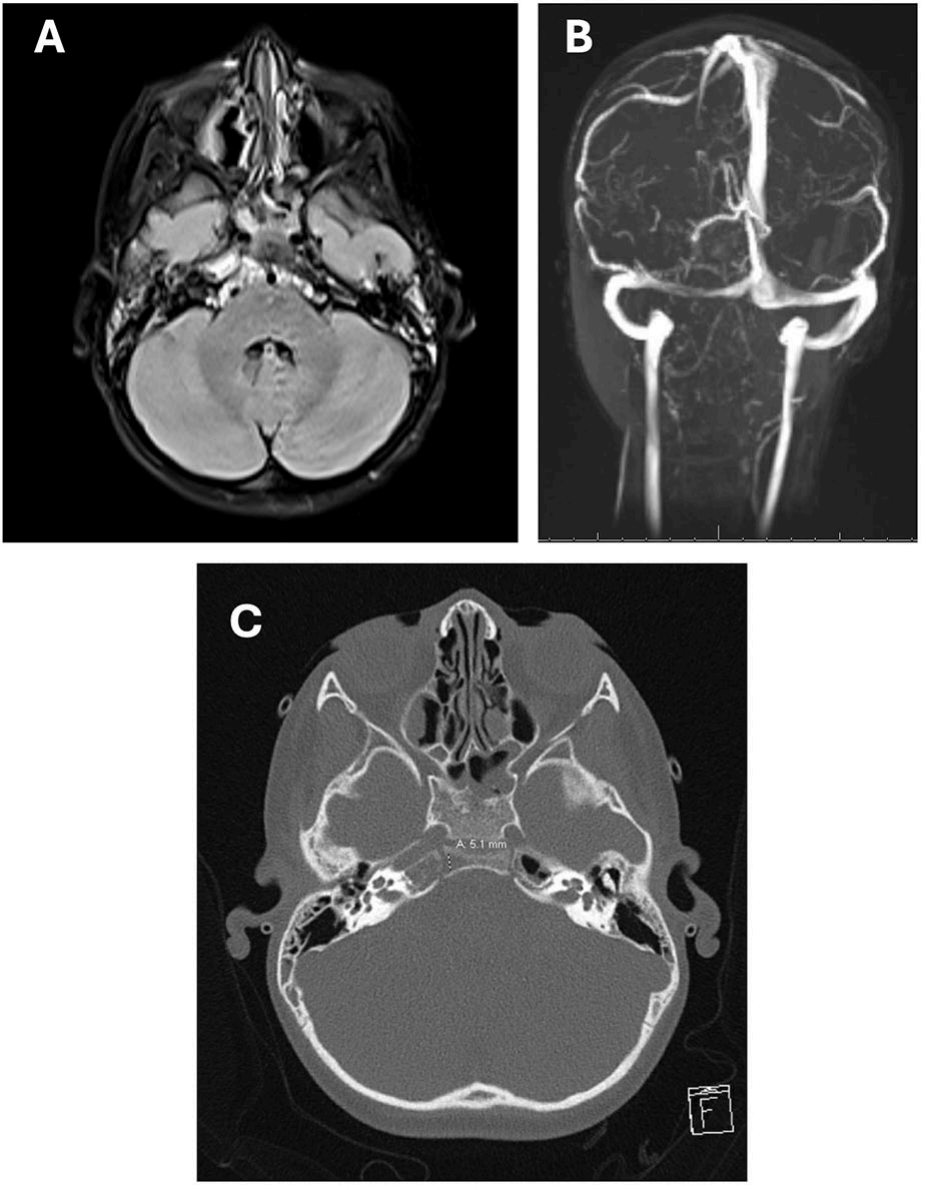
(A) MRI at follow-up week 1. There is interval improvement of the inflammatory changes involving the right petrosal-clival junction, sphenoid sinus, and right petrous apex. The small focus of diffusion restriction at the right lateral clivus, noted at admission, is no longer present. (B) There is interval improvement of the right ICA caliber likely from resolving surrounding inflammation and associated mass effect. Improved otomastoid opacification noted with some residual fluid especially at the right petrous apex. (C) CT demonstrates bony lucency within this area potentially suggestive of a small Brodie's abscess.
